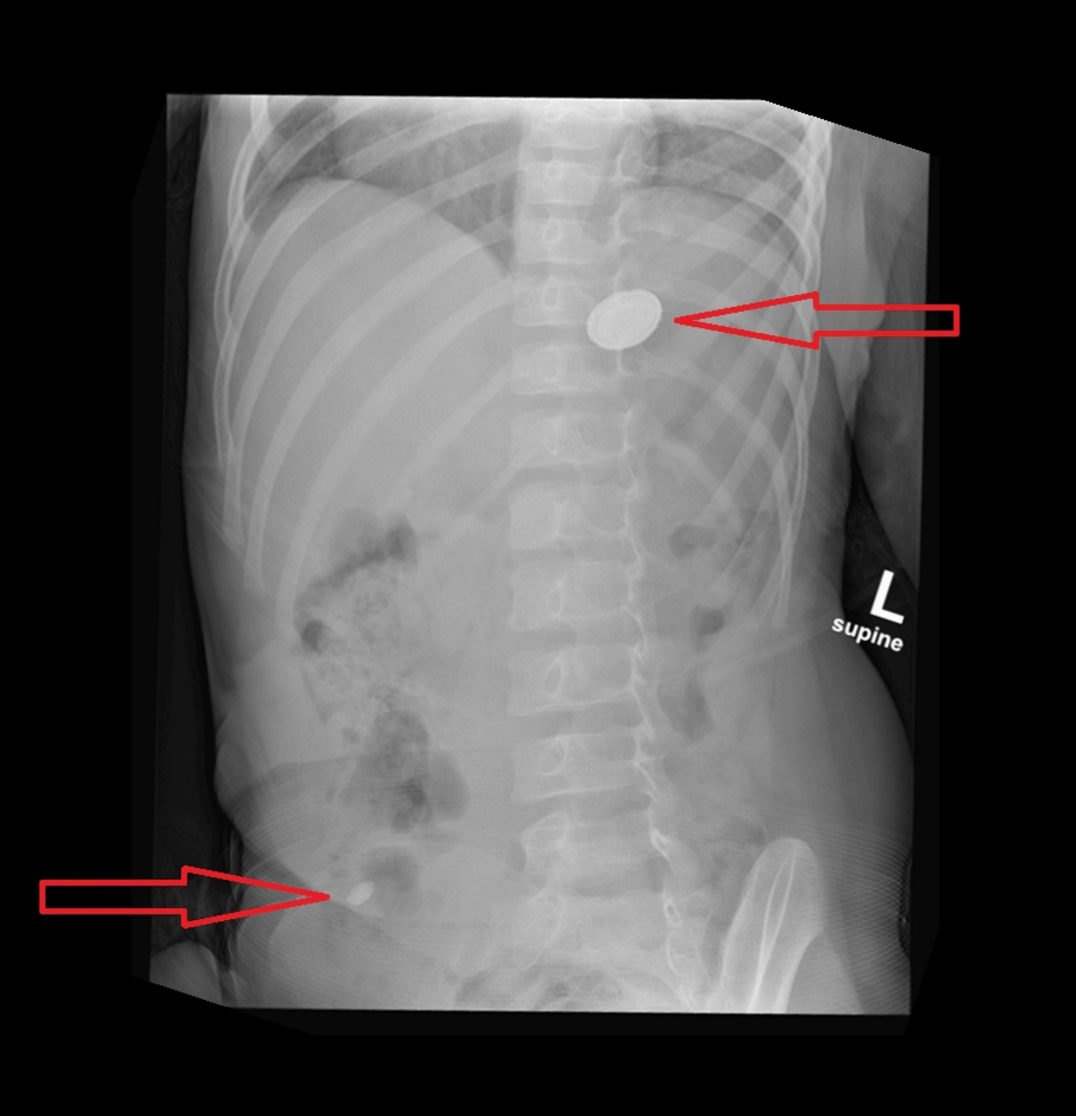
Abdominal X-ray showing two radio-opaque foreign bodies
